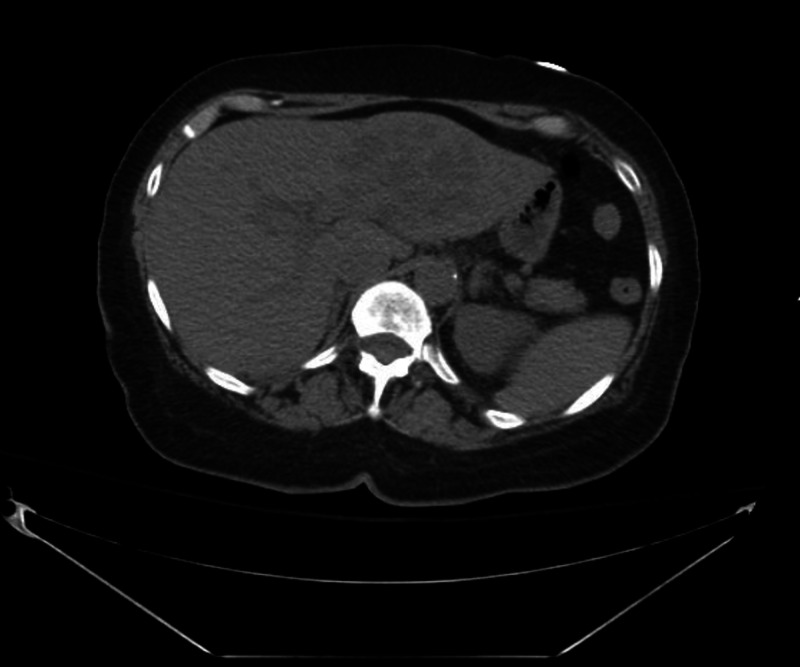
CTA/P showing a significant interval increase in the size of the heterogeneous left liver lesion, which now measures 11.5 cm in maximal dimension as compared with 7.8 cm on the prior CT scan four days earlier The lesion now has a more multiloculated appearance, highly suggestive of an abscess given the rapid interval change. There are new areas of hyperdensity along the inferior aspect of the lesion suggestive of areas of hemorrhage within the abscess. CTA/P: computed tomography arterial portography
